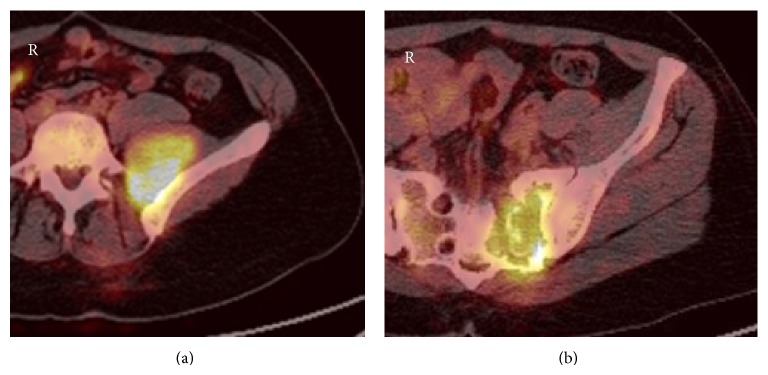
PET CT left osteolytic sacral lesion of 6.2 × 3.5 × 8.6 cm S1, S2, and S3 vertebral involvement extending to the neural foramina with very intense hypermetabolism.
